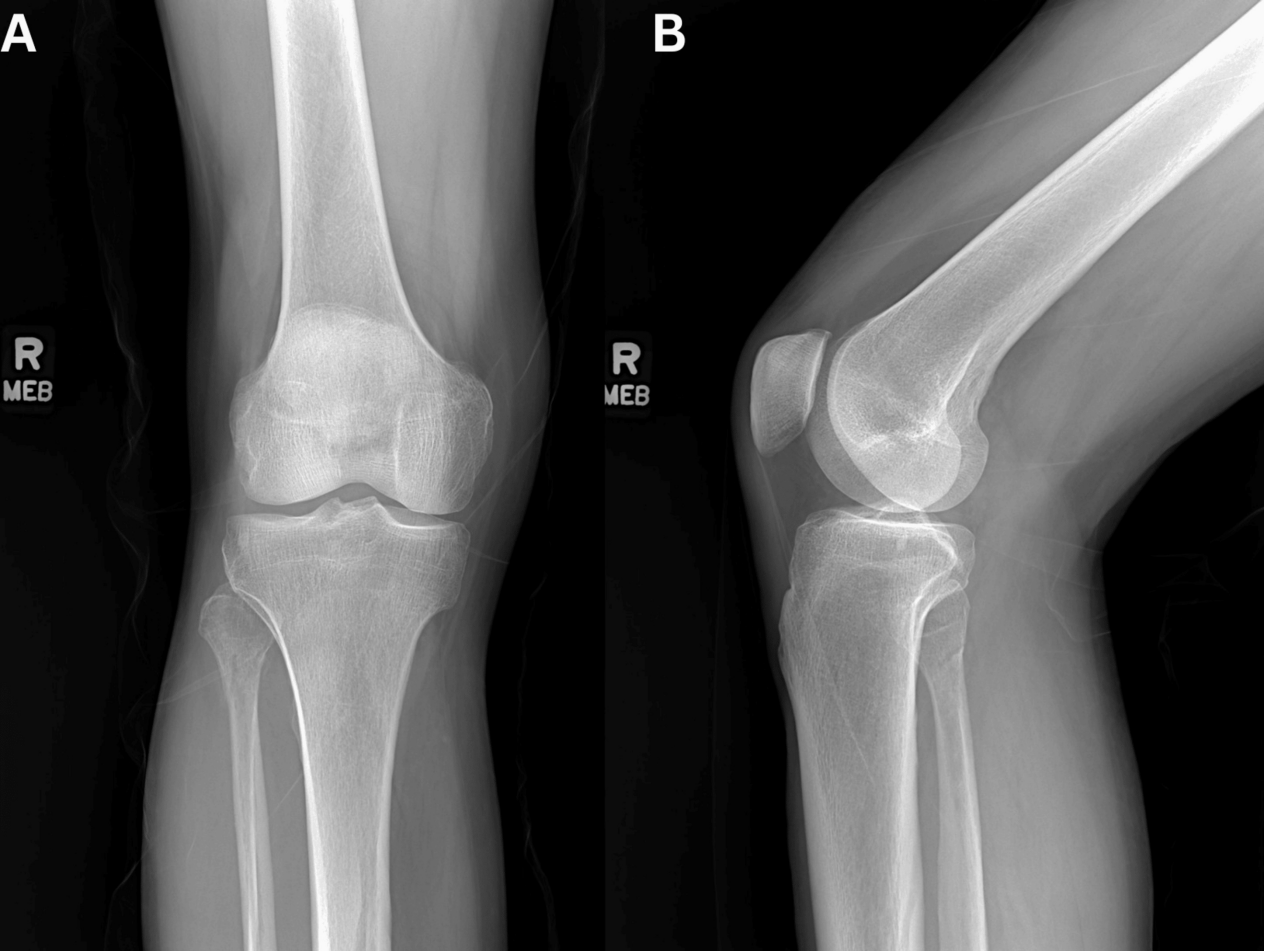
Initial X-rays of the right knee. (A) Anteroposterior and (B) lateral X-ray views of the right knee, taken approximately 24 hours after the injury, showing no fractures or abnormalities.
